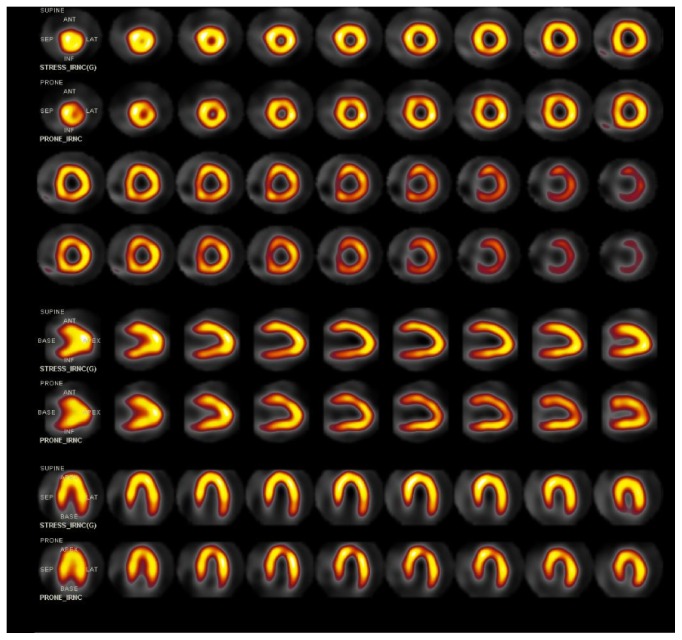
SPECT stress-only Tc-99m sestamibi myocardial perfusion imaging, demonstrating normal left ventricular perfusion. Short axis, vertical long axis, and horizontal long axis images, with images with patient supine displayed in odd rows and corresponding images with patient prone displayed in even rows.
